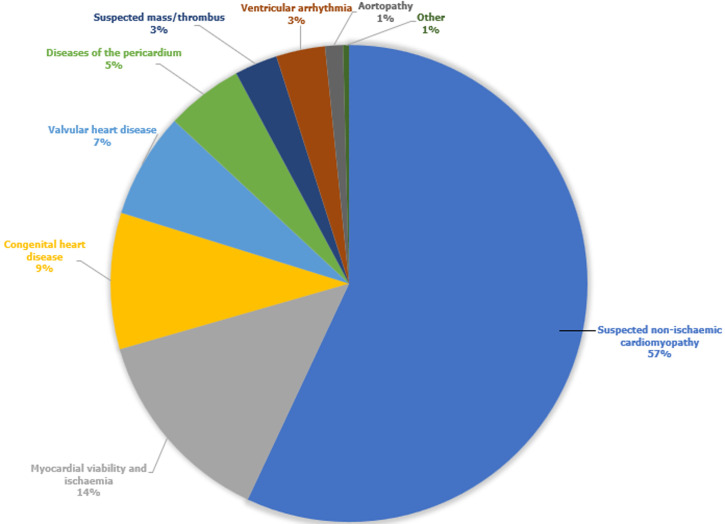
primary indications of reported clinical cardiac magnetic resonance imaging scans performed at Tygerberg Hospital, a public tertiary institution in Cape Town, South Africa from 1^st^ April 2015 to 31^st^ October 2022 (n=1403)
